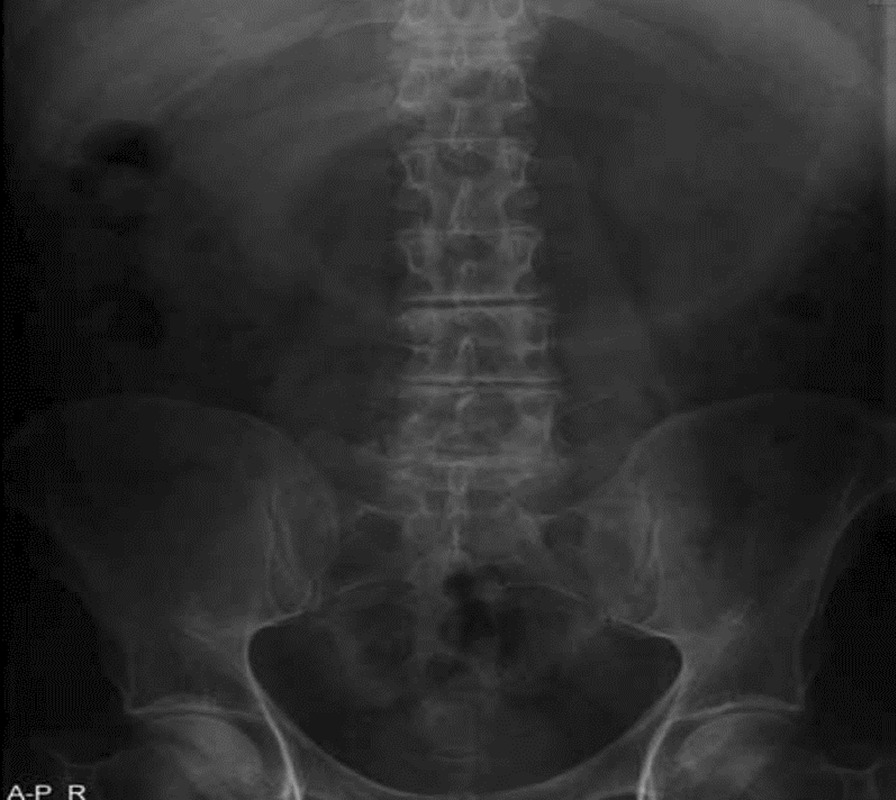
Abdominal X-ray showing distended stomach
